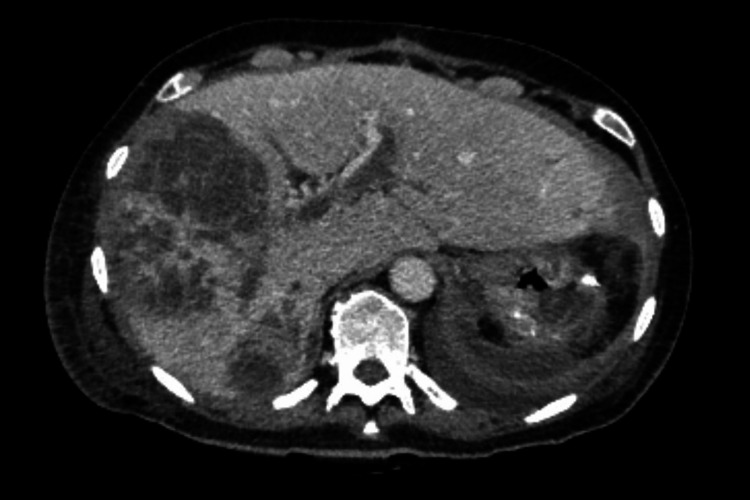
Postoperative abdominal CT scan (venous phase, axial plane): massive progression of liver metastases with neoplastic intrahepatic portal vein thrombosis and necrotic areas one month after surgery. CT: Computed Tomography
